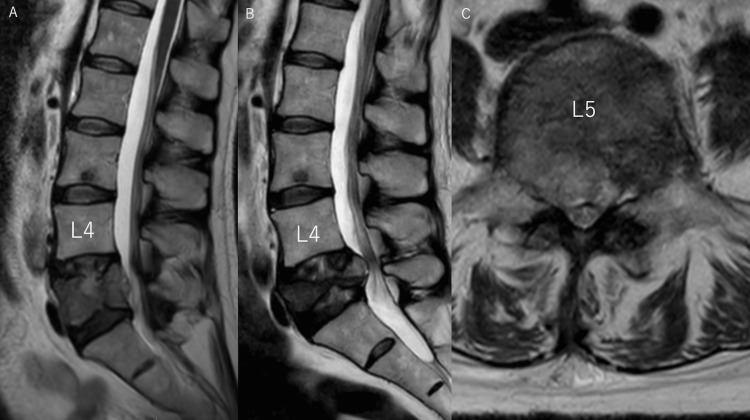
(A) T2-weighted MRI sagittal section at the initial examination. (B) T2-weighted MRI sagittal section one month after the initial examination. (C) T2-weighted MRI transverse section one month after the initial examination. A. High-intensity tumor was seen at the center of the L5 vertebral body and in the epidural region. B. The L5 vertebral body showed progressive collapse, and the high-intensity tumor protruded posteriorly, presenting spinal canal stenosis. C. The L5 vertebral body showed a significant high-intensity tumor extending the epidural space predominantly on the right side. This protrusion resulted in severe dural canal compression and intervertebral foraminal stenosis.
